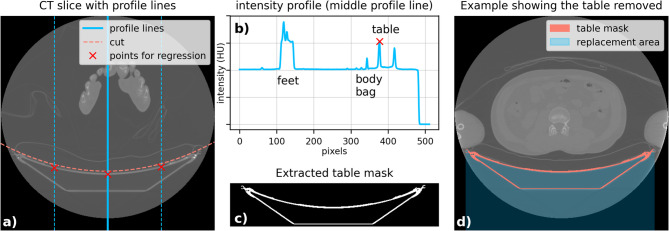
Automatic removal of the CT table: (a) Profile lines were drawn at 25%, 50%, and 75% of the image width. (b) The intensity profile was analyzed to find the table edges. (c) Quadratic regression was used to isolate the table, and (d) a mask replaced the table area with − 1000 HU (air).
